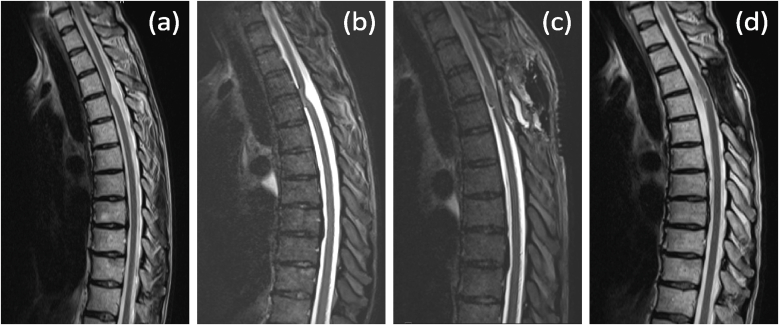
MRI of the thoracic spine in T2 sequence: a)demonstrates a kinked (C-shaped) spinal cord at the Th3/Th4 level 3 months before surgery; b) immediately preoperatively, showing signs of increased kinking of the spinal cord; c) directly postoperatively, confirming reduction of the hernia; d) at the 4-month follow-up, confirming resolution of the hernia.
